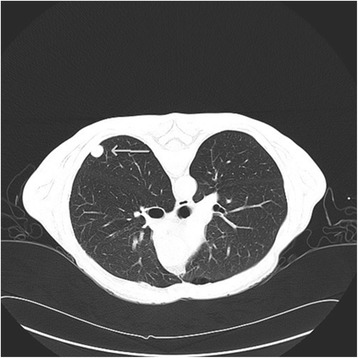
Chest CT scan of Patient showing lung lesion indicated by arrow. The lung biopsy was consistent with moderately differentiated SCC
